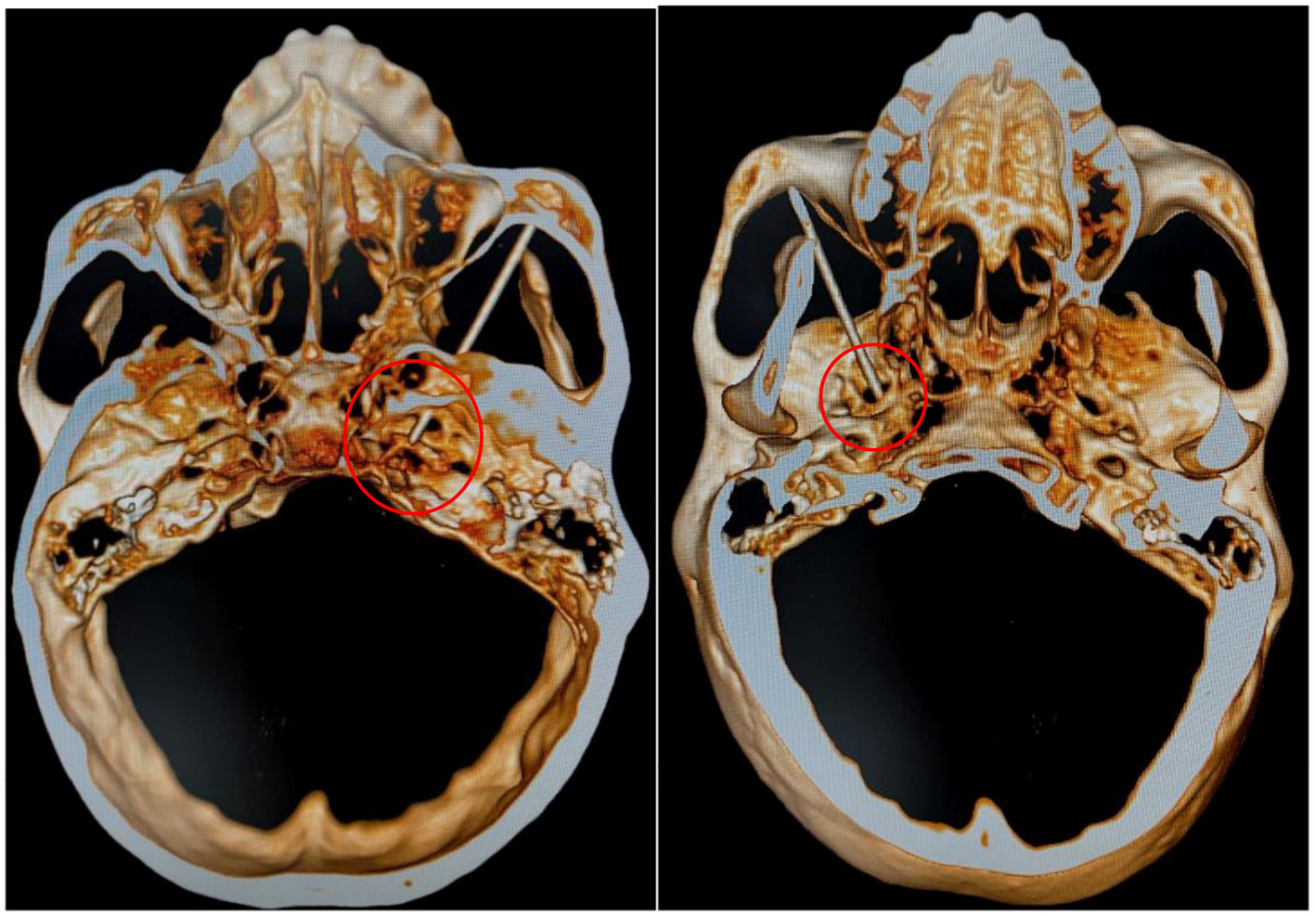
Under CT guidance, the precise position of the needle tip was confirmed. The puncture needle was advanced to the optimal depth within the foramen ovale, with meticulous efforts to avoid the mandibular nerve.
